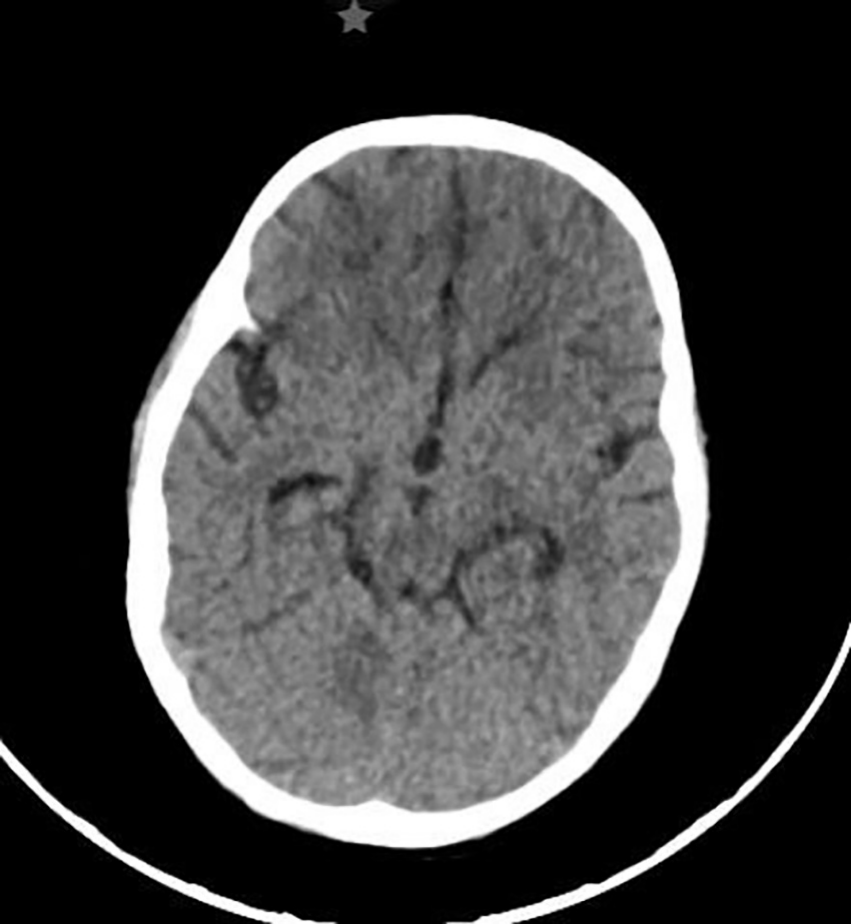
Cranial Computed Tomography (CT) Scan with Evidence of Hematomas. The CT scan revealed hypodense lesions in the frontal region, consistent with bleeding that progressed toward the convexity. There was no displacement of the midline.
